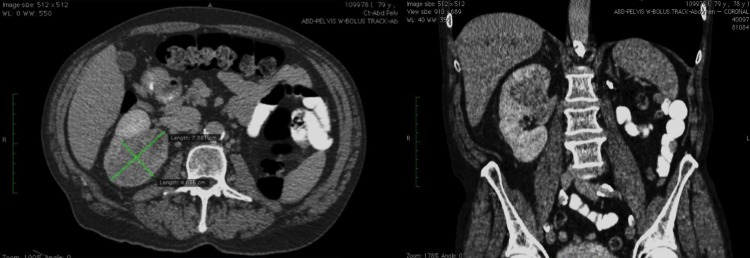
Pretreatment axial-contrasted CT scan of the abdomen and pelvis demonstrating right renal mass. Coronal view of 7.4 cm right upper pole renal-cell carcinoma extending caudally involving >50% of solitary kidney.
